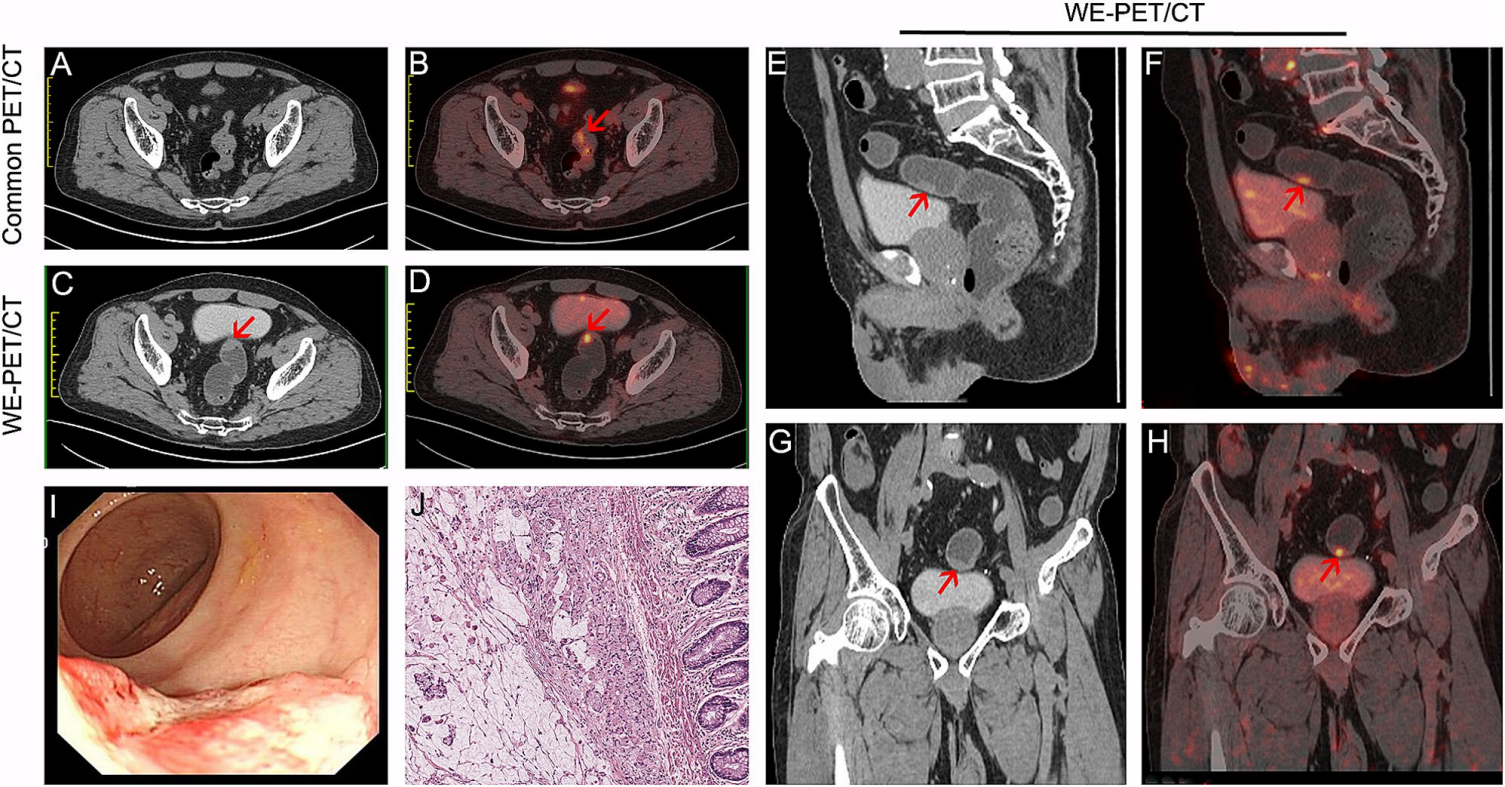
A 74-year-old male patient with cancer screening. Uneven ^18^F-FDG uptake in the sigmoid colon and a collapsed bowel was shown on common PET/CT (A-B). On WE-PET/CT, the tumour was clearly shown as focal ^18^F-FDG uptake with correlative wall thickening (C-H, red arrow). Colonoscopy (I) and pathological analysis (J) confirmed T1 mucinous adenocarcinoma
